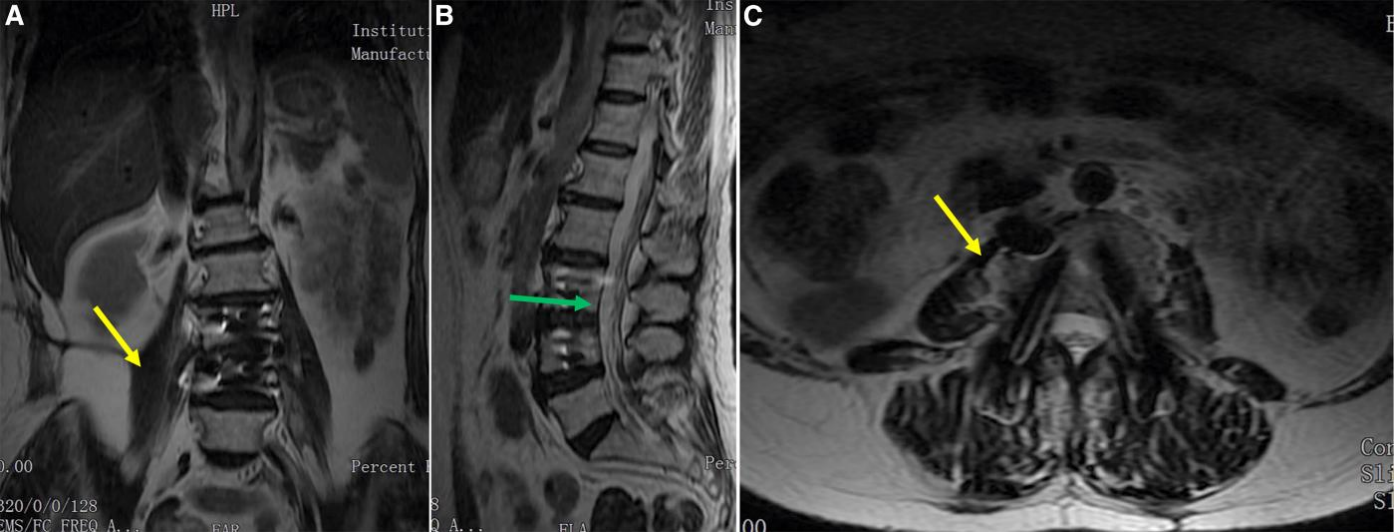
Magnetic resonance (MR) images at the 12-month follow-up. (A–C) Coronal, sagittal, and axial MR images indicate that the psoas abscess (yellow arrow) and epidural abscess (green arrow) are cured.
